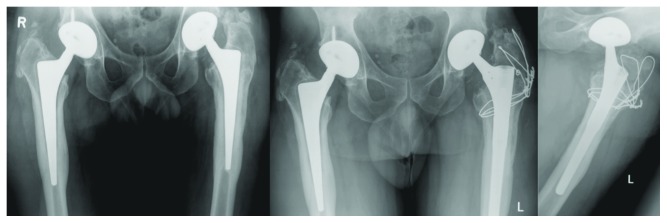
Radiograph a.p. view with loosening of the left femoral stem, radiograph a.p. and axial view of first revision.
